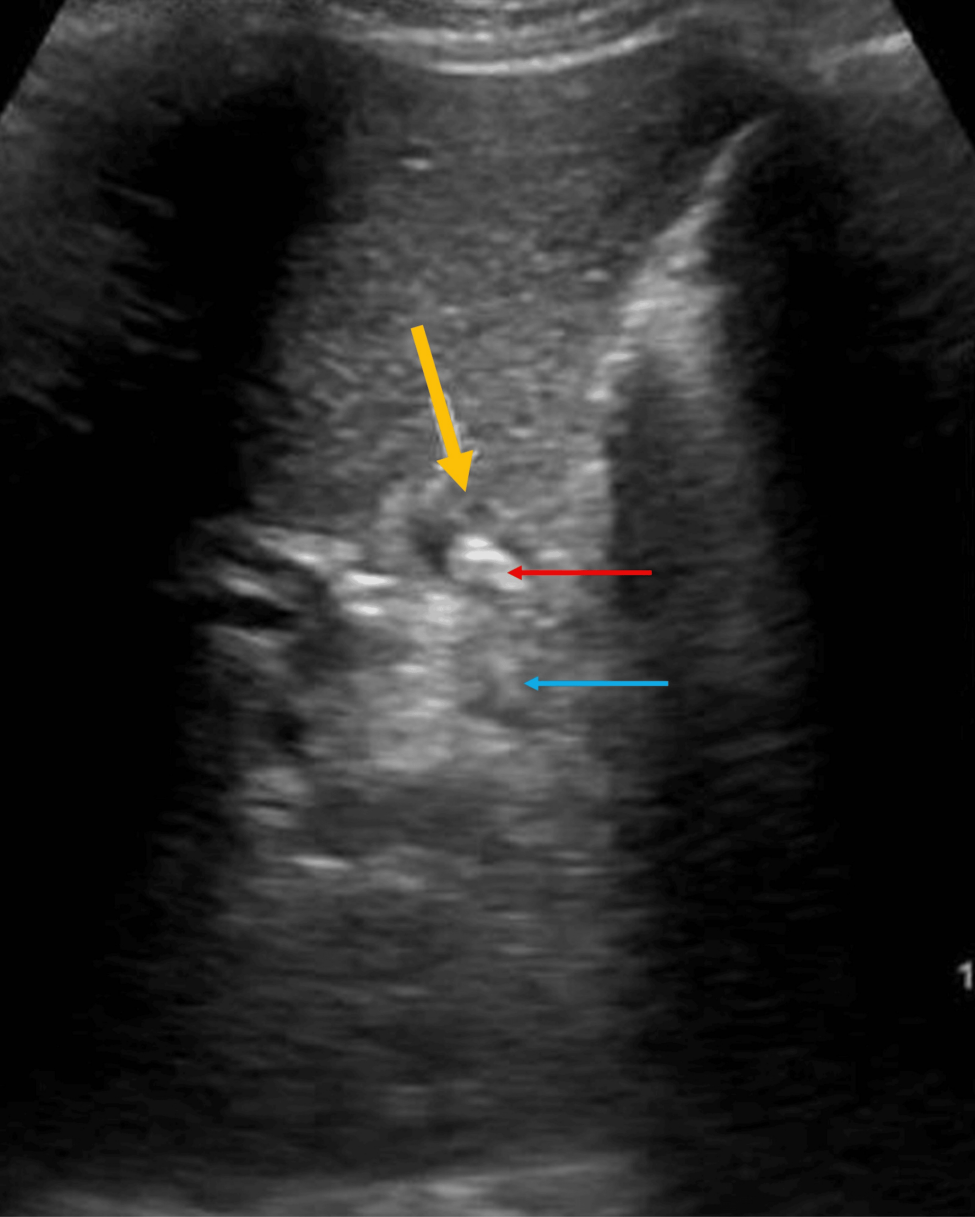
Abdominal ultrasound showing residual gallbladder stump or dilated cystic duct remnant (yellow arrow) with echogenic foci (red arrow) casting posterior acoustic shadow (blue arrow), suggestive of calculus.
